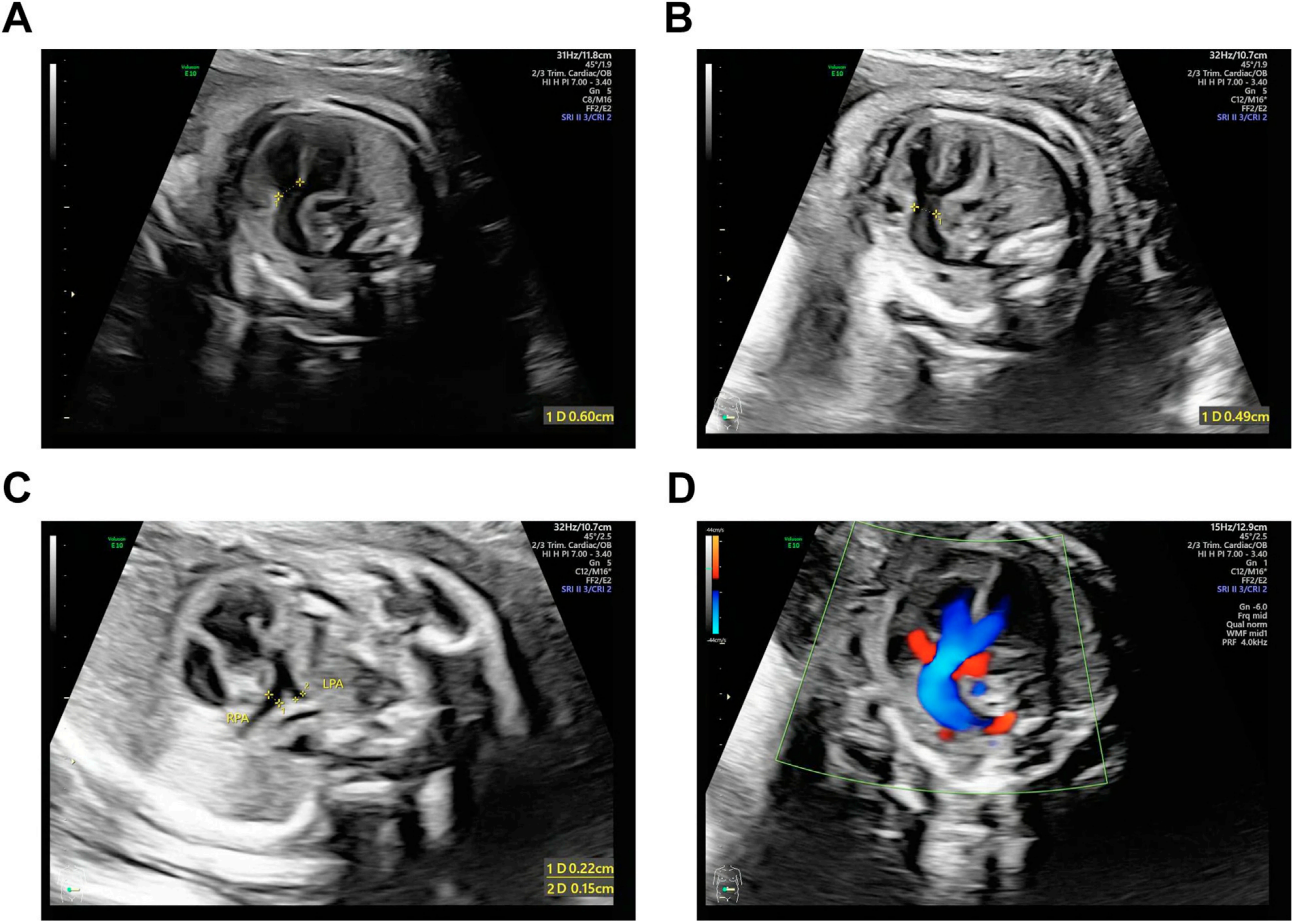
Representative prenatal ultrasound image of a fetus with an *FGD5* variant. (A) Interrupted aortic-septal continuity: 0.60-cm defect. (B) Aortic luminal diameter: 0.49 cm. (C) Pulmonary artery stenosis: left PA 0.15 cm and right PA 0.22 cm (D) Systolic right-to-left VSD shunting causing aortic dilation and reduced pulmonary arterial flow.
